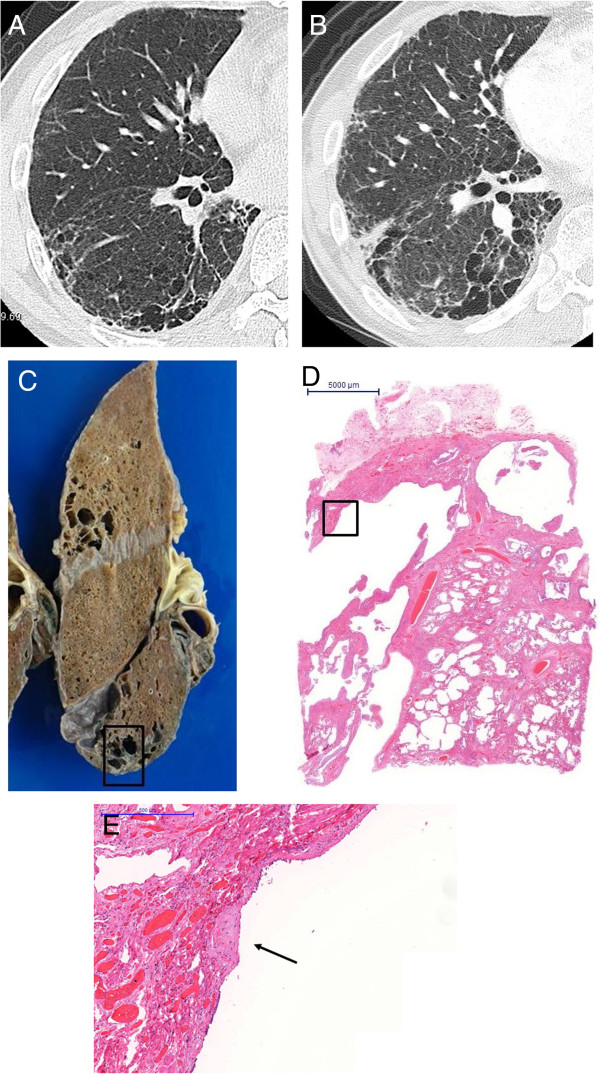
Images of a CPFE patient without lung cancer who died from right heart failure owing to deterioration of pulmonary hypertension. (A) CT scan performed 6 months prior to death; view of the right lower lobe showing TWCLs and traction bronchiectasis with reticulation on the pleural predominance. (B) CT scan performed within 1 month of death showing enlargement of TWCLs and simultaneous progression of reticulation despite smoking cessation. (C) Gross photo of the right lower lobe showing TWCLs (square). (D) Paramount view showing TWCLs apposed to honeycombing in the right lower lobe; corresponds to the CT scan (Figure 4-B) and gross photo (Figure 4-C). (E) High-power view of the square lesion in Figure 4-D showing fibroblastic focus (arrow) in the fibrous walls of the TWCLs.
